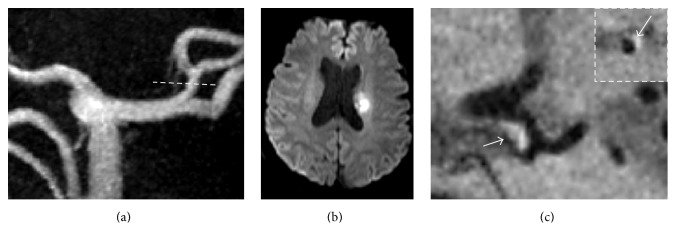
A 56-year-old man with right-side weakness and dysarthria. (a) MRA shows no stenosis on the left MCA; (b) the diffusion-weighted image shows a hypertensive lesion in the left lenticular nucleus with rostral extension to the coronal radiate; (c) the T1-weighted SPACE postcontrast images show apparent eccentric wall thickening (arrow) in close proximity to bifurcation ipsilateral to the infarction on both the long axis and the short axis of the vessel.
